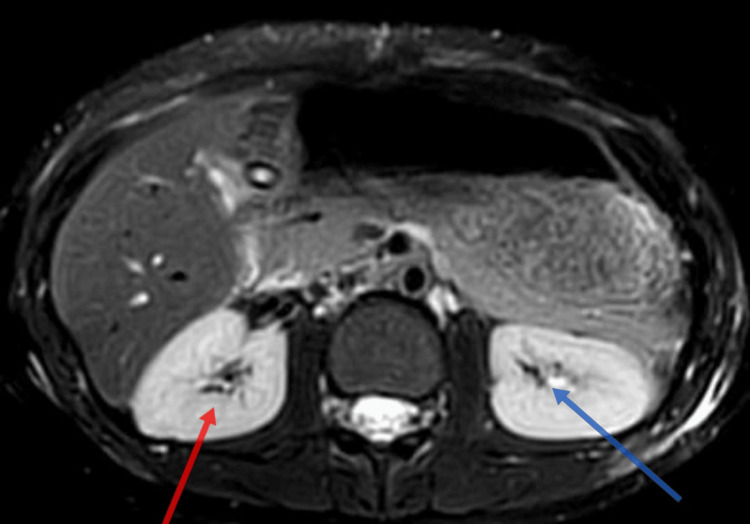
Magnetic resonance image showing right kidney laceration Red: right kidney; Blue: left kidney.
